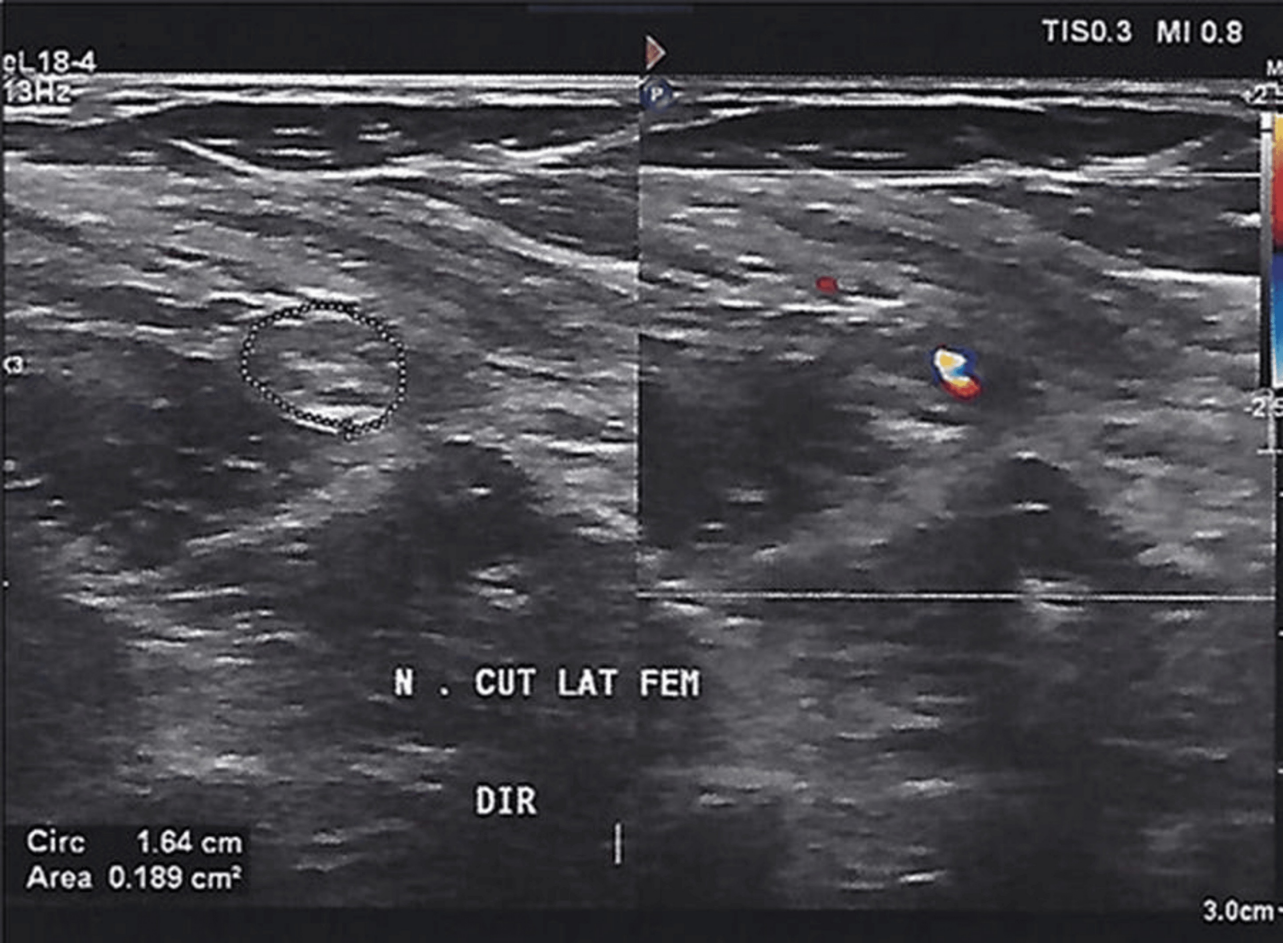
Right LFCN presented as a hypoechogenic and thick fusiform image (highlighted), scanned above the inguinal ligament. Focal loss of fascicular pattern is also observed. Doppler US showed peri- and intraneural vascularization increase (reference values for cross-sectional area: 0.9-1.5 cm2). LFCN: lateral femoral cutaneous nerve; US: ultrasound
